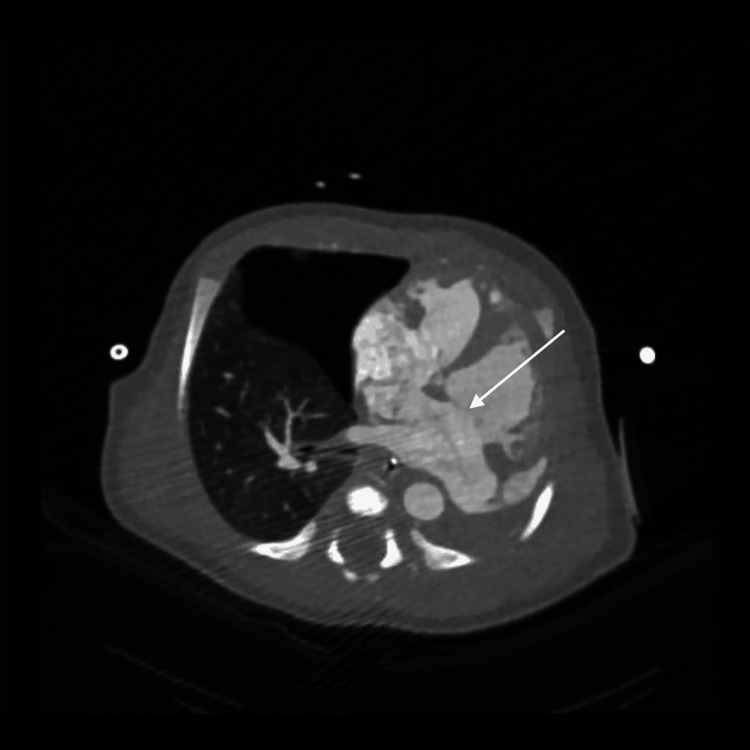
Axial computed tomography image revealing a communication across the interatrial septum consistent with an atrial septal defect (white arrow)
